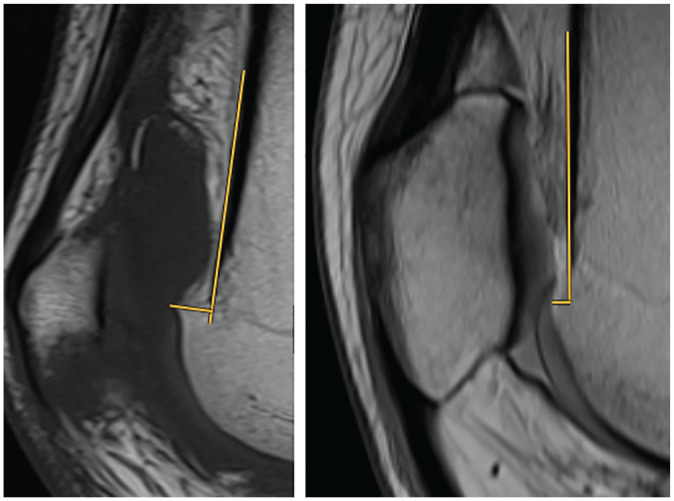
Supratrochlear spur height measurement on midsagittal magnetic resonance imaging, defined as the maximal height of the osseous trochlear floor in relation to a tangent of the anterior cortex of the distal femur. The left image shows a large supratrochlear spur height of 7.5 mm, whereas the right image shows a small anterior translation of the trochlear floor of 2.1 mm.
